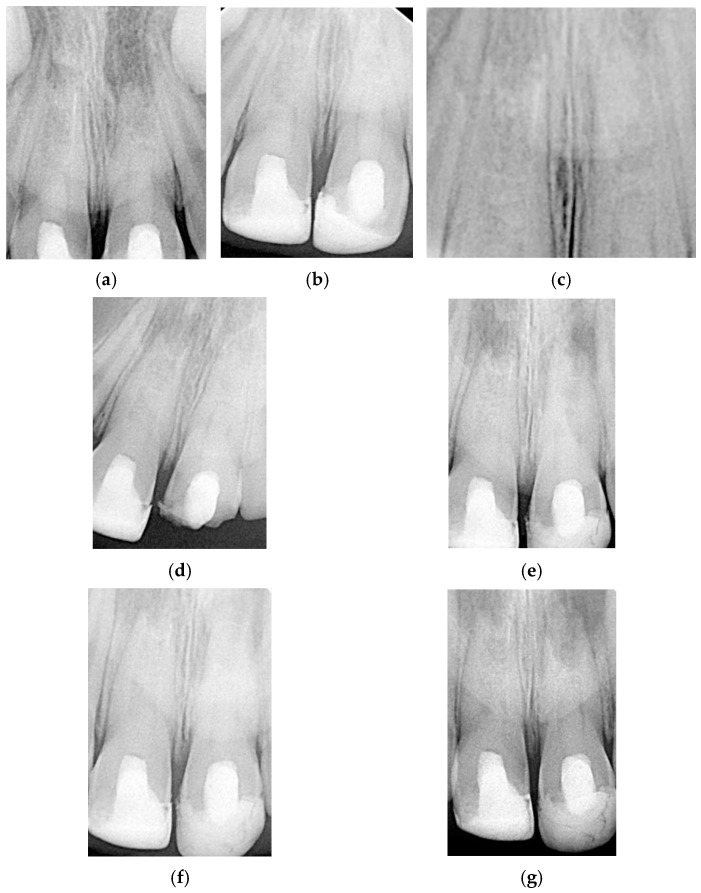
Clinical Case 2. Periapical radiograph taken at (a) 6-month follow-up, (b) 1-year follow-up, (c) 2-year follow-up, (d) 3-year follow-up, (e) 4-year follow-up, (f) 5-year follow-up, and (g) 6-year follow-up.
